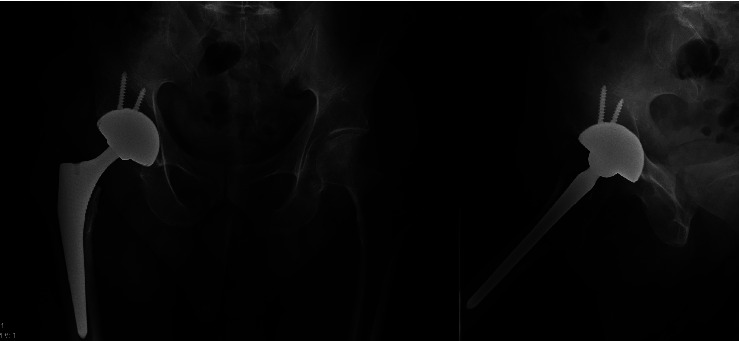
Postoperative AP and lateral radiographs demonstrating implantation and appropriate positioning of the novel DM implant used in primary total hip arthroplasty.
